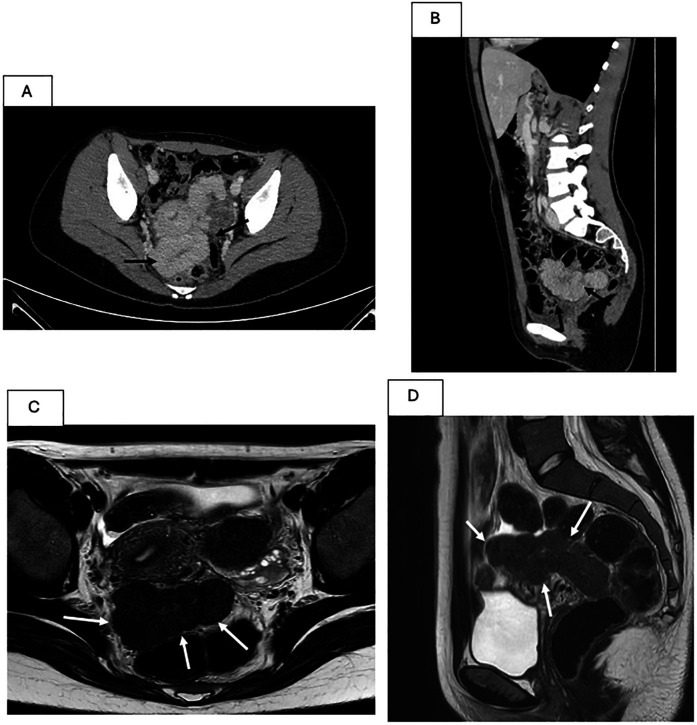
CT and MRI aspect of the pelvic splenosis. (A,B) CT scan. A serpiginous formation with dimensions of 120 × 60 × 55 mm, with homogeneous contrast enhancement in the portal-venous phase, is indicated by the black arrows in axial (A) and sagittal (B) plane. (C,D) MRI. Multilobulated formation with regular margins and confluent pseudonodular components with elongated morphology (axial dimensions of 10 × 4.7 cm and a craniocaudal extension of approximately 6.3 cm) is indicated by the white arrows, located between the uterus and the rectum in axial T2-weighted (C) and sagittal T2-weighted (D) images.
